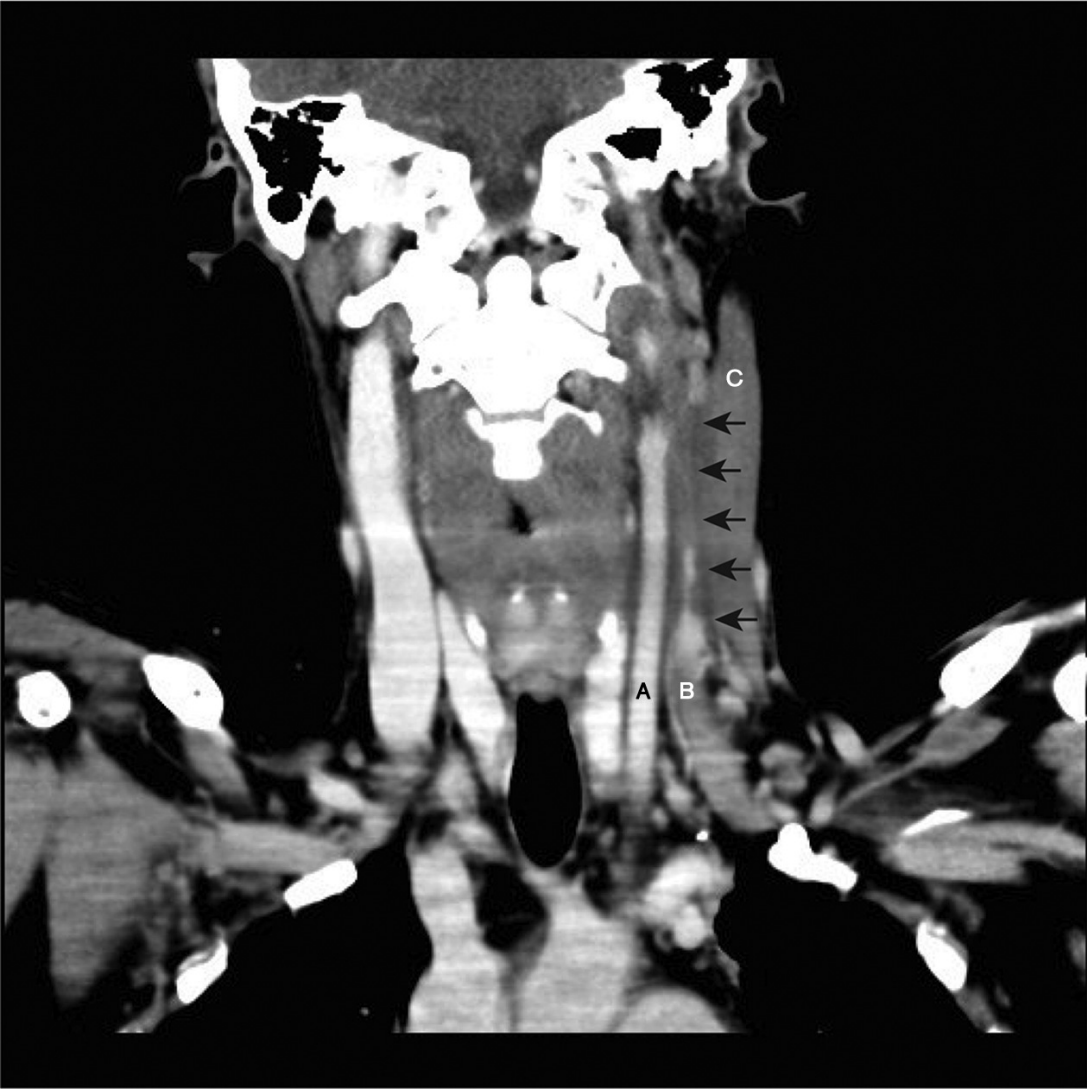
Post-contrast CT scan of the neck showing the left common carotid artery (A) and left internal jugular vein (B). Arrows indicate the extent of the thrombosis and the filling defect of the left internal jugular vein at the level of the thyroid cartilage, extending superiorly to the base of skull. There was also fusiform thickening of the left sternocleidomastoid muscle (C) without a discrete collection.
